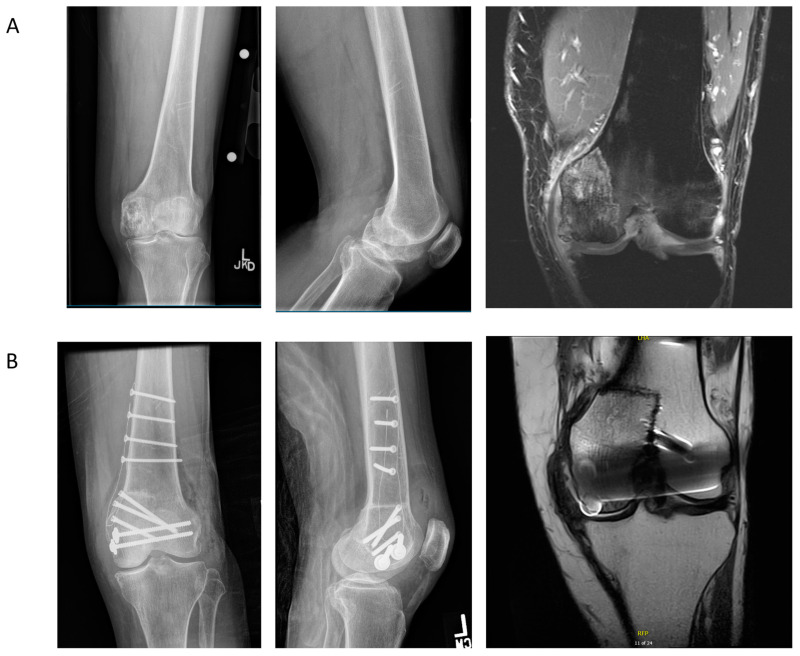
(A) Pre-operative anteroposterior (AP) and lateral radiographs of the distal femur and T1-post-contrast fat-saturation coronal magnetic resonance (MR) image of the knee, demonstrating a grade 2 chondrosarcoma of the medial femoral condyle. (B) The 12-month post-operative AP and lateral radiographs and proton density coronal MR image of the knee, demonstrating interval hemicondylar resection and osteoarticular allograft reconstruction with a carbon fiber plate and supplemental screw fixation. Minimal susceptibility artifact from the plate is noted with comparatively higher artifact from the metallic screws.
